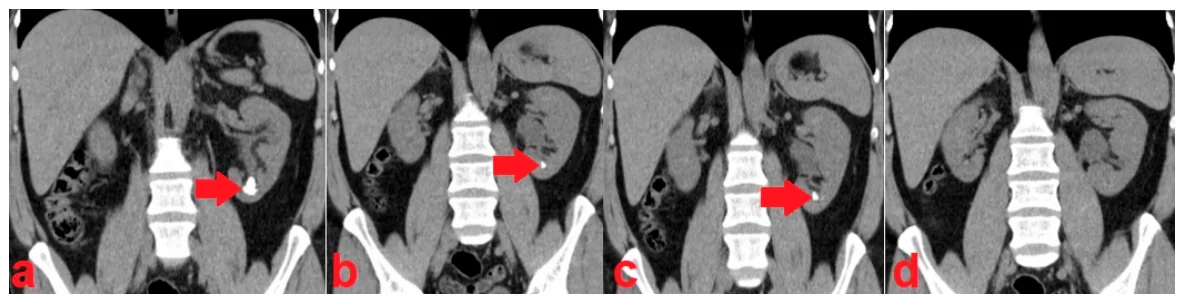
Serial non-contrast computed tomography (NCCT) images of a patient who achieved documented spontaneous fragment passage: (a) Preoperative NCCT demonstrating an isolated left lower-pole calculus measuring 17.0 mm in maximum diameter (arrow); (b) Baseline NCCT obtained at 3 months postoperatively (study time zero) confirming eligibility with a persistent 5.0 mm residual stone fragment (arrow); (c) Persistence of the calculus (arrow) on NCCT performed at 12 months postoperatively; and (d) Final NCCT at 18 months postoperatively confirming complete spontaneous stone clearance (terminal stone burden: 0.0 mm^2^).
